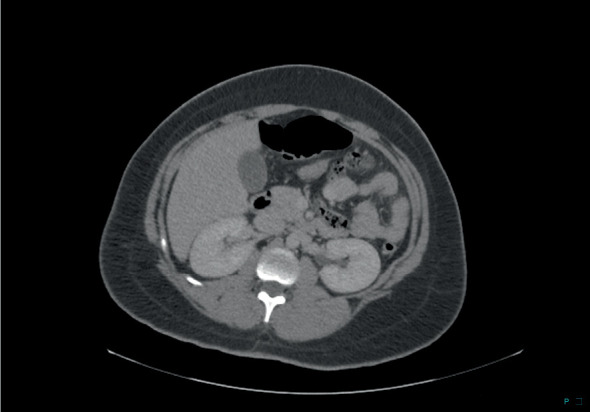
CT image shows an absent spleen in the left upper quadrant.
